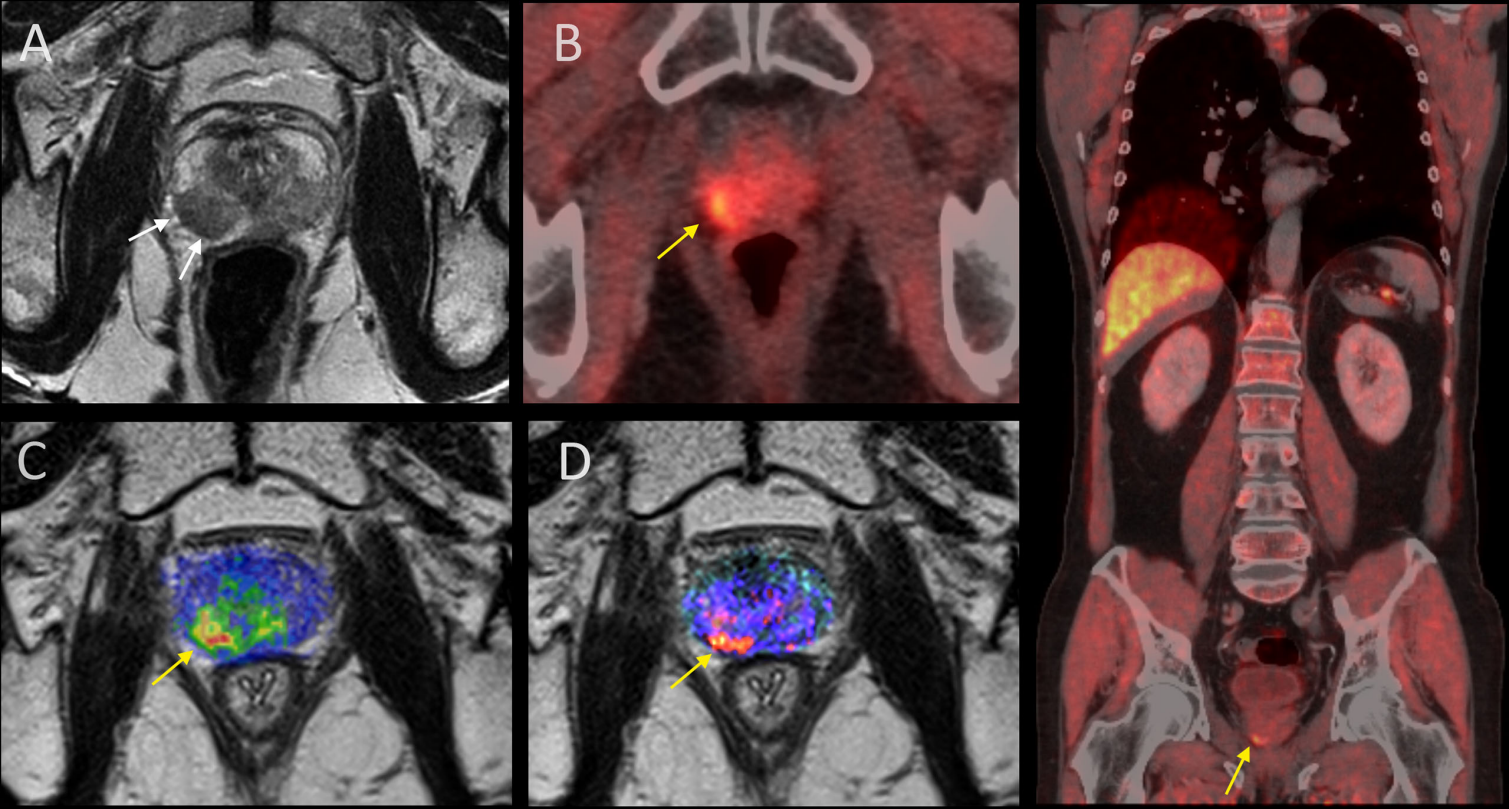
Primary and radio-recurrent prostate cancer at imaging. (A) Primary tumor at T2W MRI (white arrows). (B–D) Recurrent tumor (yellow arrow) at FACBC PET/CT, diffusion weighting overlaid on T2W MRI, and dynamic contrast-enhanced MRI overlaid on T2W MRI. To the right: Whole-body FACBC PET/CT to prove true local recurrence only (yellow arrow).
